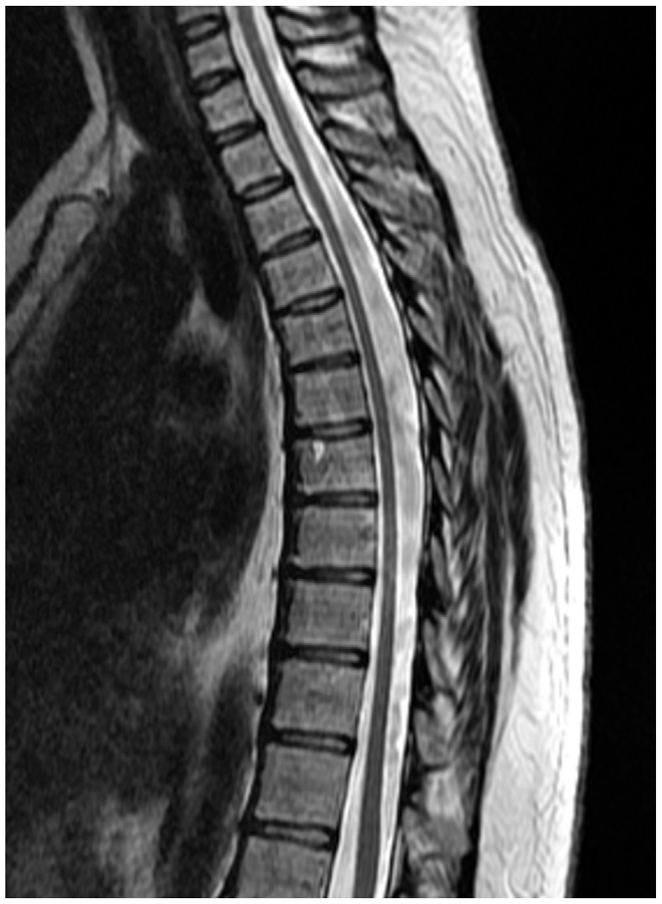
Magnetic resonance imaging of the thoracic chord (0–72 weeks). Clinical improvement was observed despite the marked atrophy of the thoracic cord.
